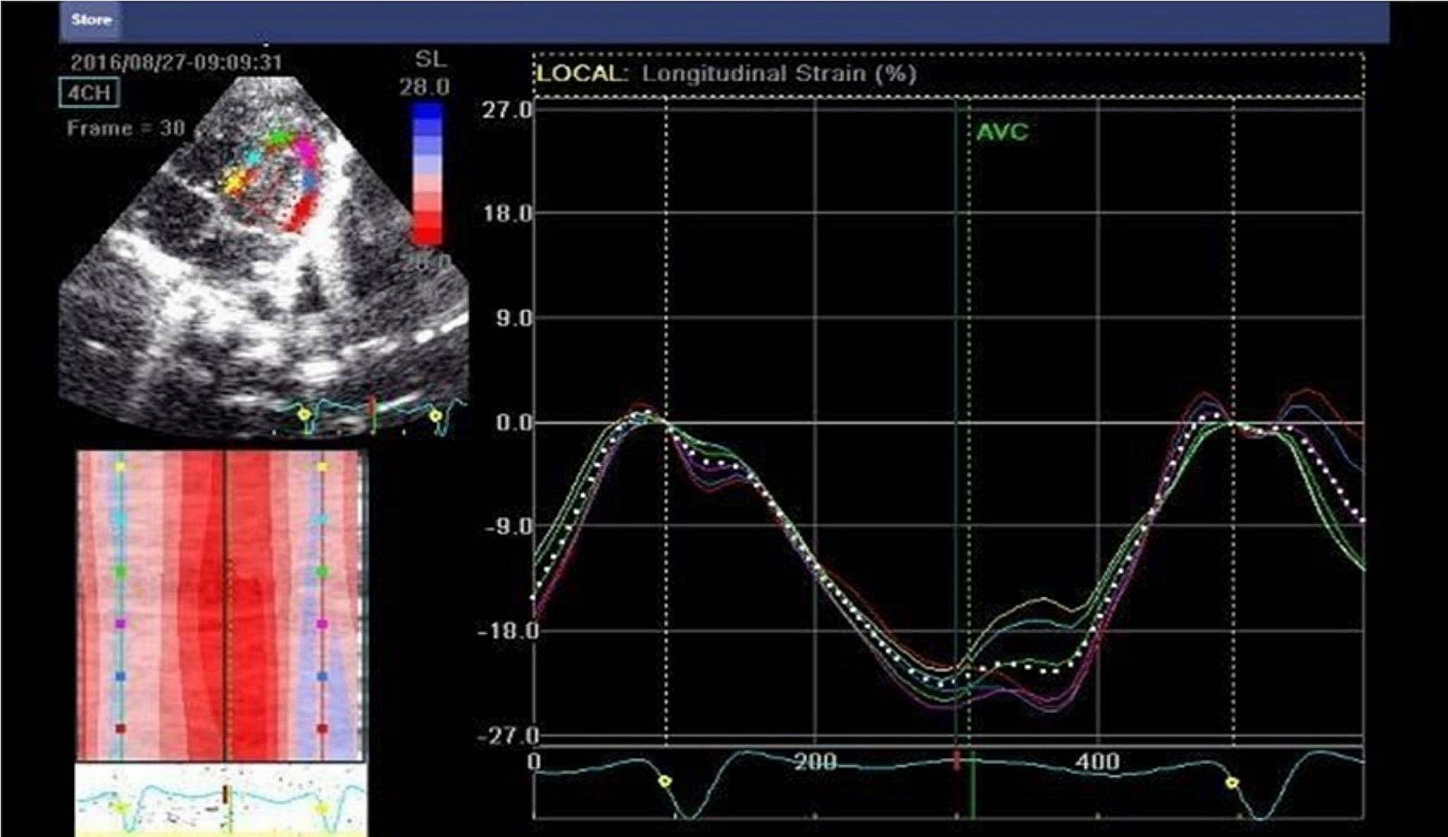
Deformation imaging of LV in apical 4ch view
